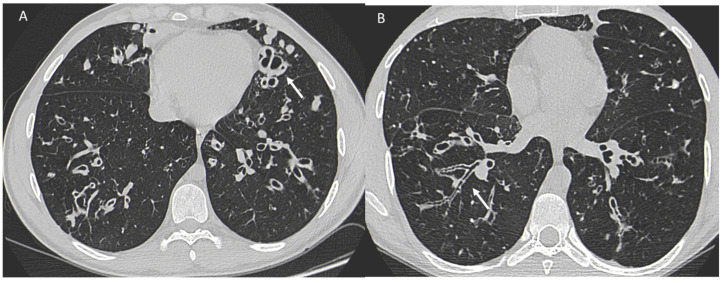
A woman and a man with CF with different and higher anatomo-structural involvement at HRCT in women with CF compared with men. (A) Diffuse cystic-varicoid bronchiectasis, with diffuse wall thickening and mucous plug (white arrow) in a young woman patient with cystic fibrosis. (B) Bronchiectasis with thickened walls (white arrow), especially in the medium-lower pulmonary lobes in a male patient with cystic fibrosis.
